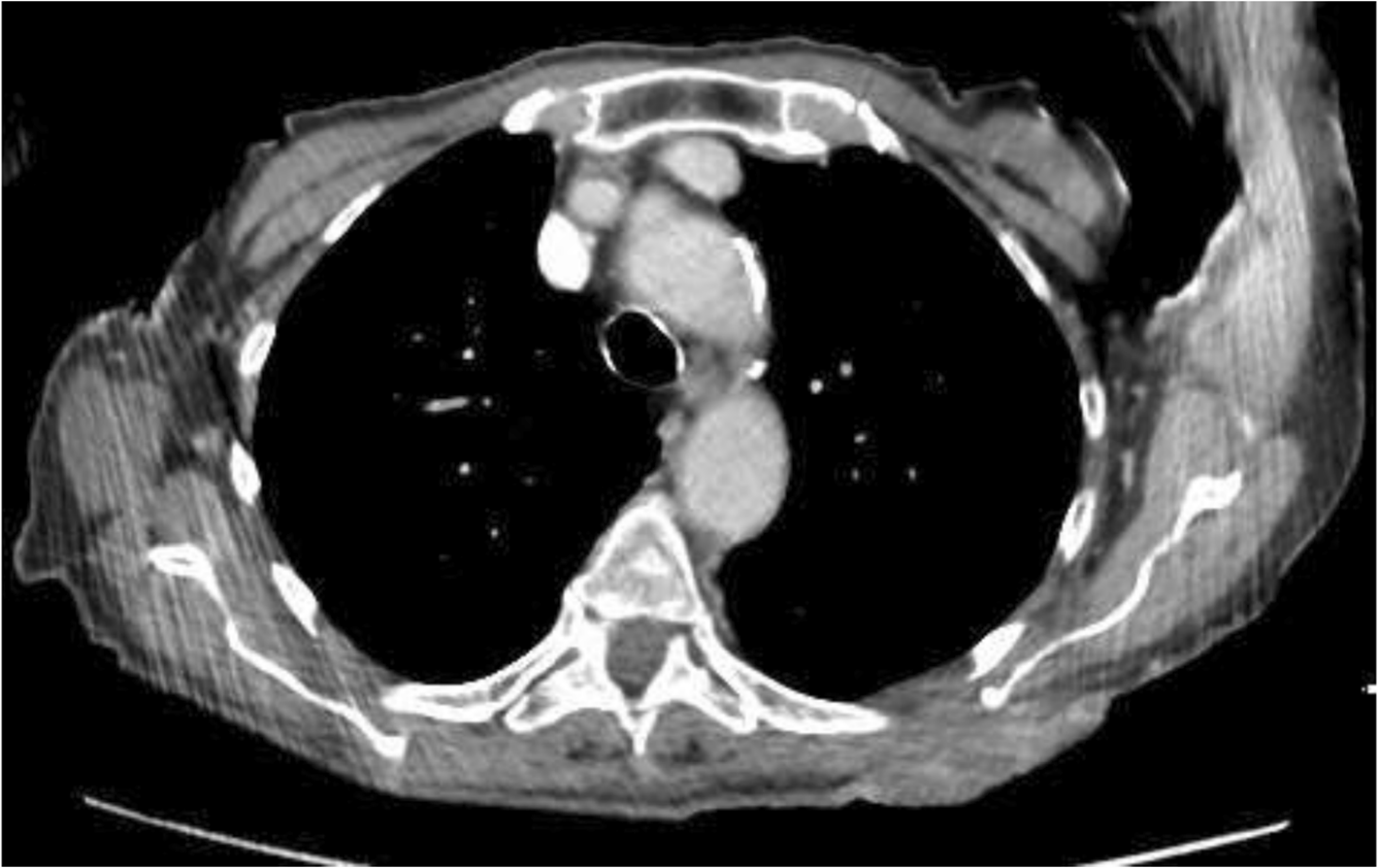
Thoracic computed tomography in axial section showing superficial dorsal lesion with no invasion of the scapula at depth
